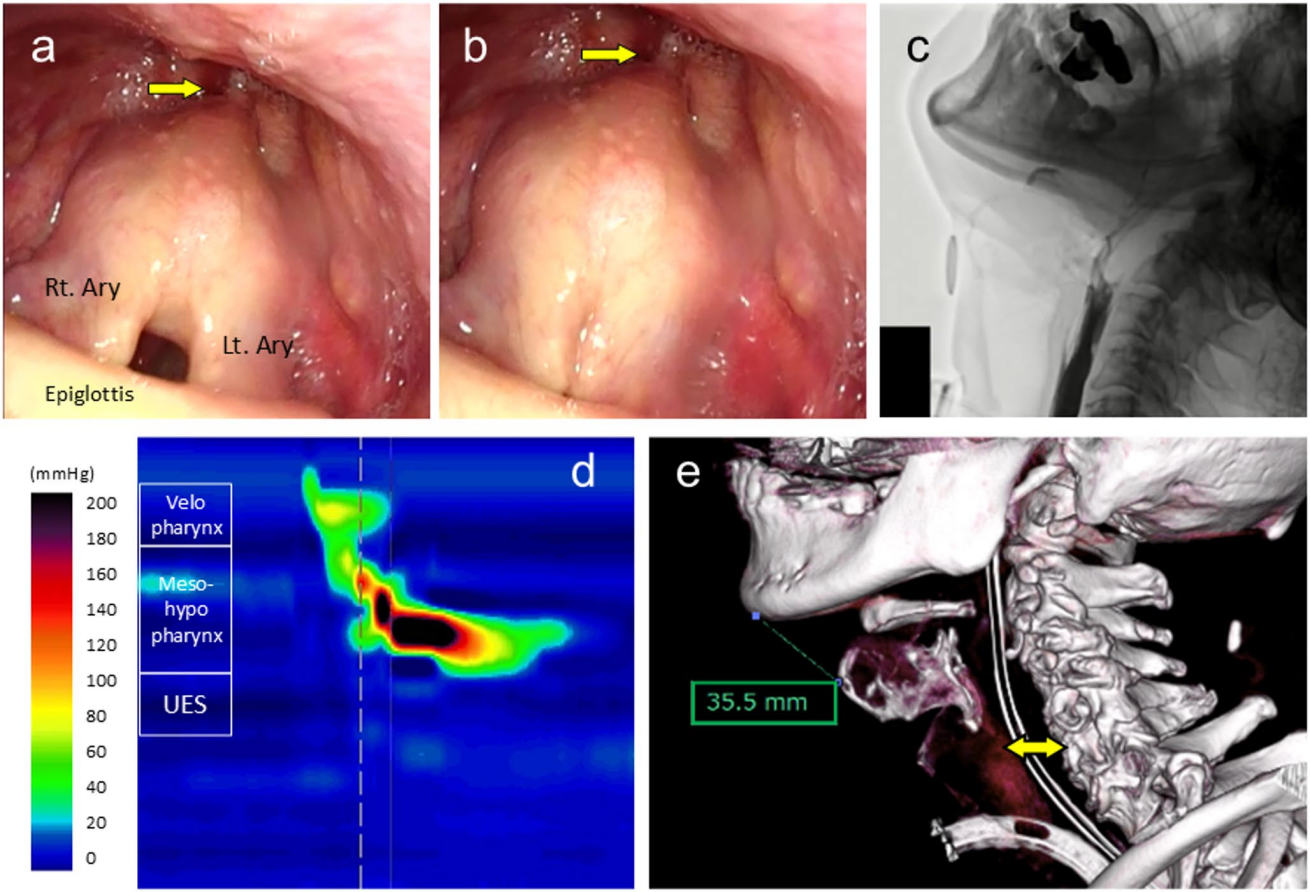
Postoperative examinations. (a, b) anterior-superior traction of the bilateral arytenoid cartilages (Ary) and visualization of the upper esophageal sphincter (UES, yellow arrows) during inspiration (a) and phonation (b). (c) videoflouroscopic swallowing study using 5 mL of thin liquid contrast agent at 60° postural angle. (d) postoperative high-resolution manometry revealed sustained opening of the UES. (e) cervical CT scan showed anterior-superior displacement of the thyroid cartilage and reduced thyromental distance (35.5 mm), with widened UES opening (double-headed yellow arrow)
